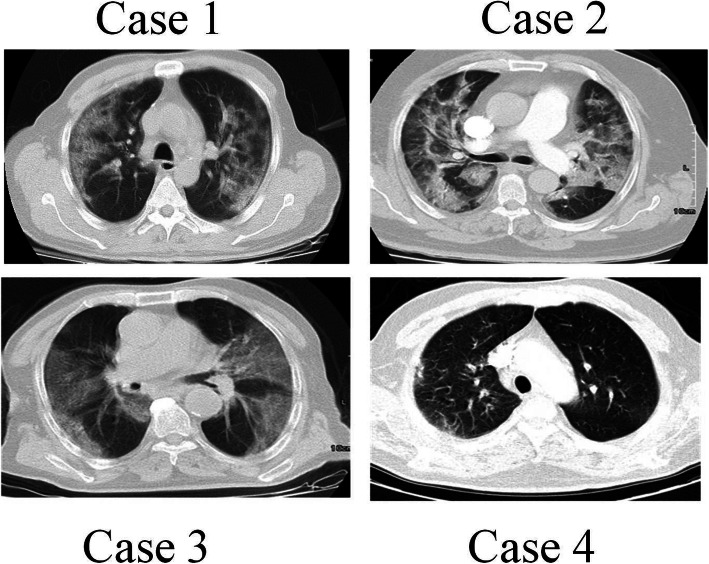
Axial non-contrast-enhanced image of computed tomography (CT) scan of the chest, showing the COVID-19 infection
